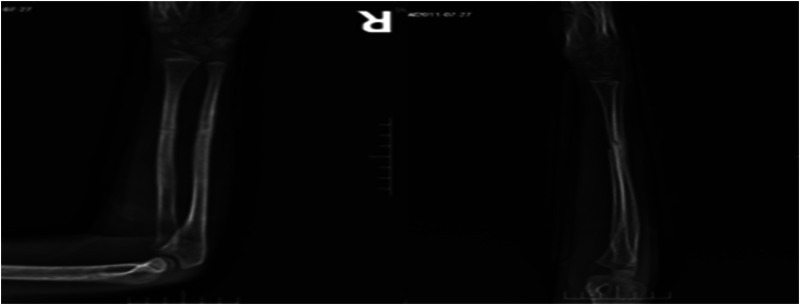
X-rays after 2 months of reset.
